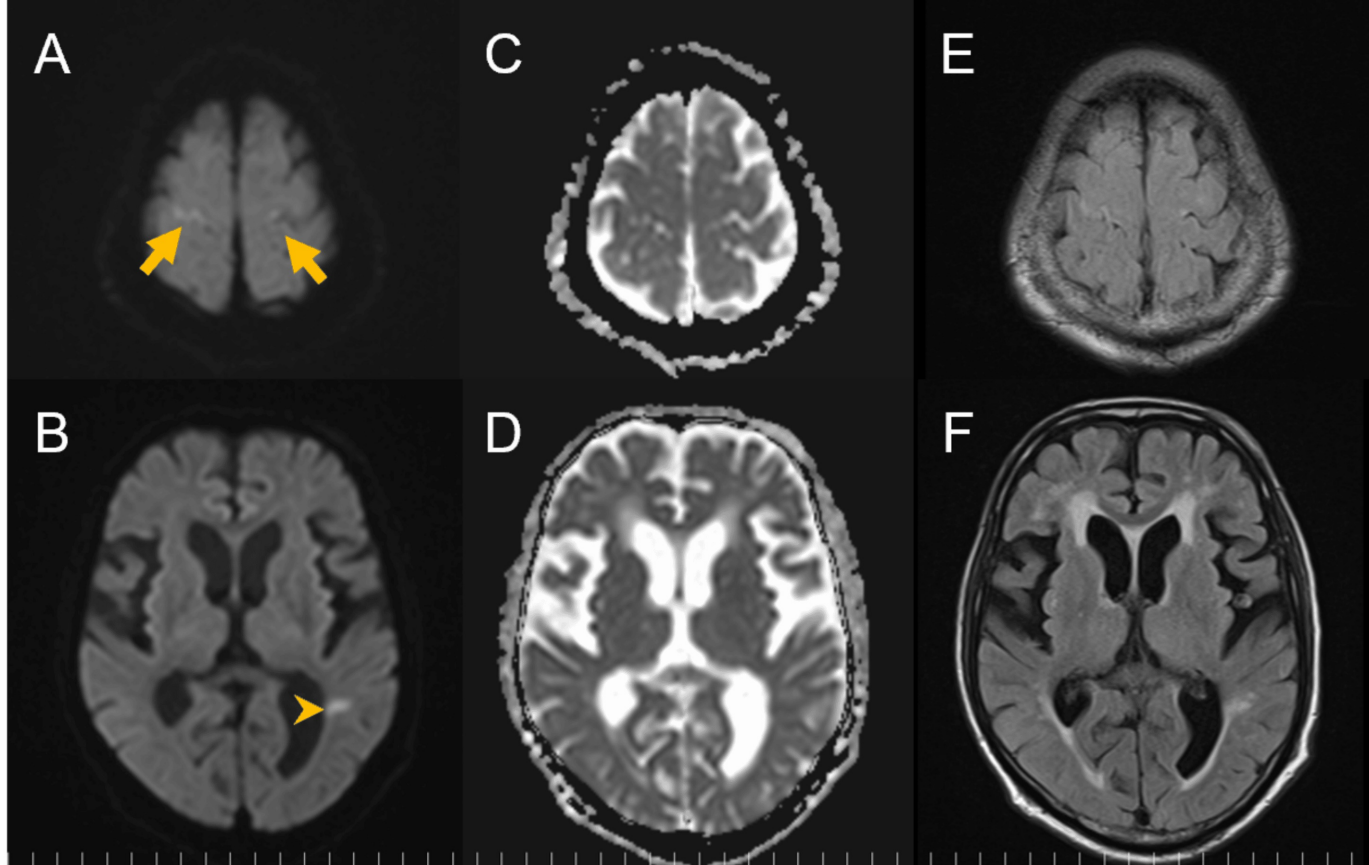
Brain MRI three days before the onset of altered mental status (A, C, E) The frontal cortex (arrow) that was later recognized as abnormal shows slightly high signal intensity on DWI, decreased ADC, and slightly high signal on FLAIR, but was overlooked at this stage. (B, D, F) A small lesion (arrowhead) in the left parietal subcortical area shows high signal intensity on DWI, reduced ADC, and high signal on FLAIR. This lesion was suspected to be ischemic but was considered unrelated to the patient's hypobulia. ADC, apparent diffusion coefficient; DWI, diffusion-weighted imaging; FLAIR, fluid-attenuated inversion recovery
